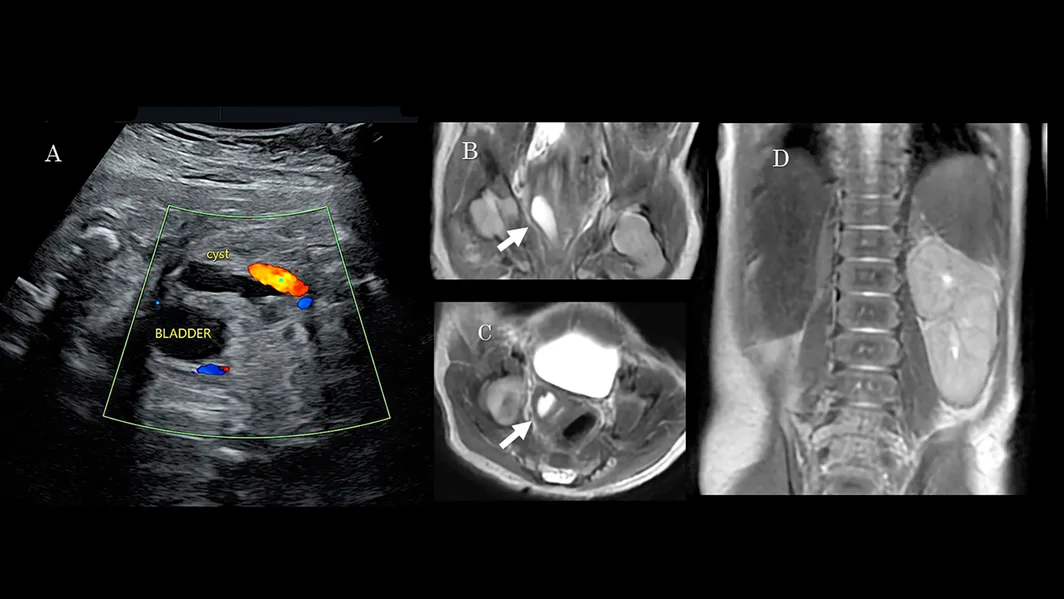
Fetal ultrasonography showing a unilocular cyst adjacent to the bladder (A). Pelvic magnetic resonance imaging obtained at 11 days of age. T2‐weighted coronal (B) and axial (C) images demonstrating a markedly dilated fluid‐filled cavity, indicative of obstructed hemivagina associated with uterus didelphys. Ipsilateral renal agenesis is evident (D).
